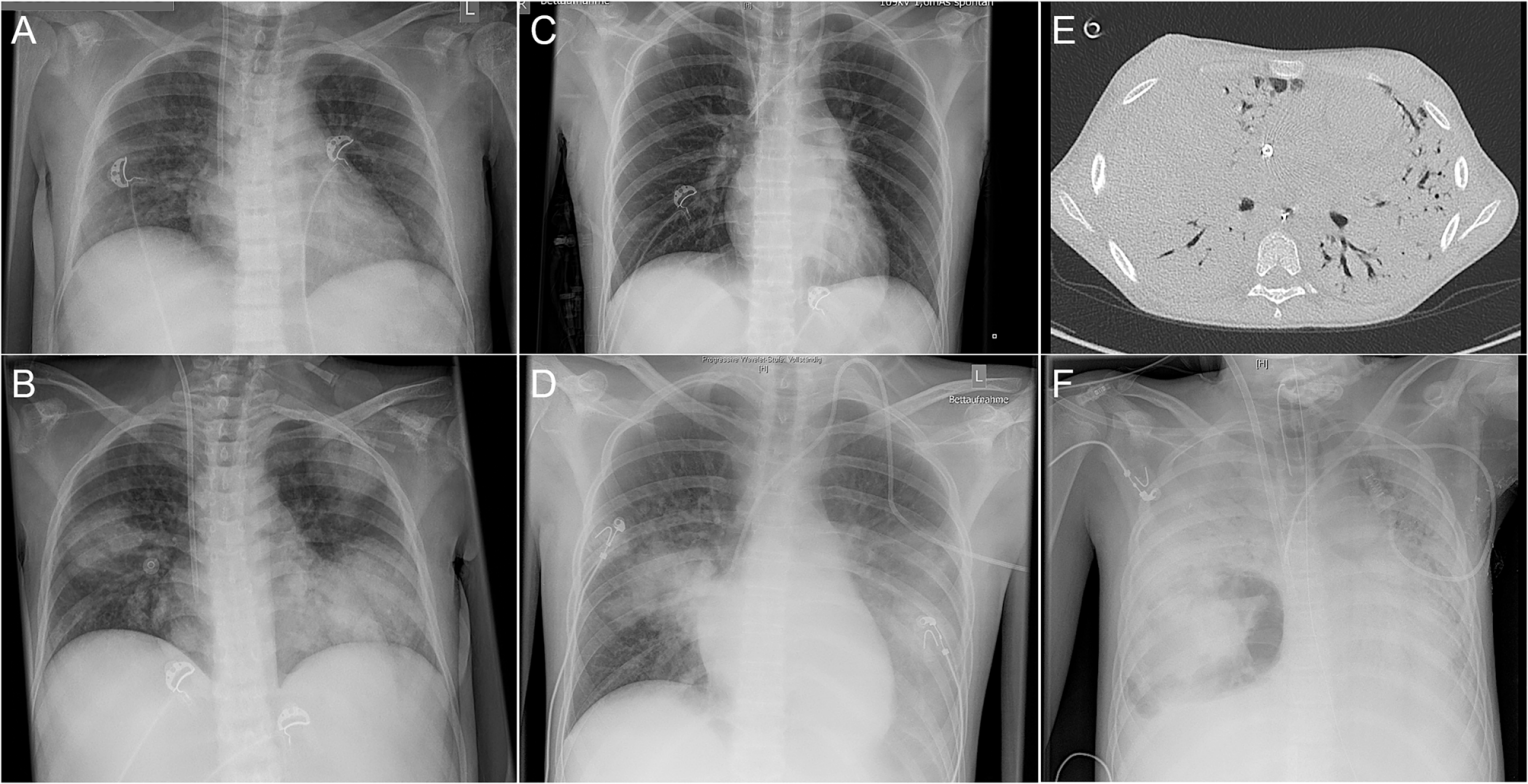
Radiographic findings in three patients with *S.maltophilia* infection and pulmonary hemorrhage. (A, B) 15-years old girl post allogeneic HCT for aplastic anemia (patient 4). (A) Normal chest x-ray at day +1 following allogeneic HCT; (B)
